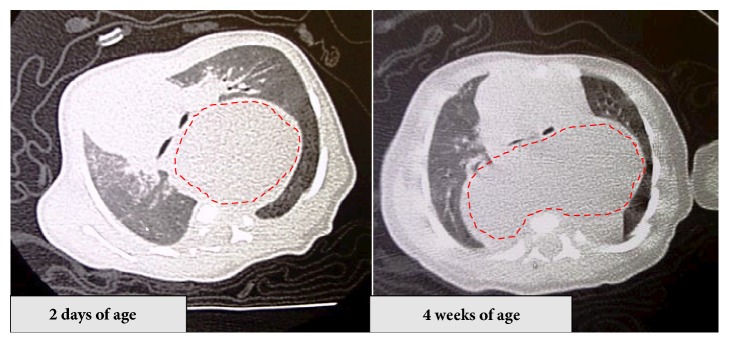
Four weeks after delivery, the neonate was admitted because of respiratory distress due to mass effect. Computed tomography scans (right panel: age 2 days, left panel: age 4 weeks) show enlargement of the mediastinal cystic lesion.
